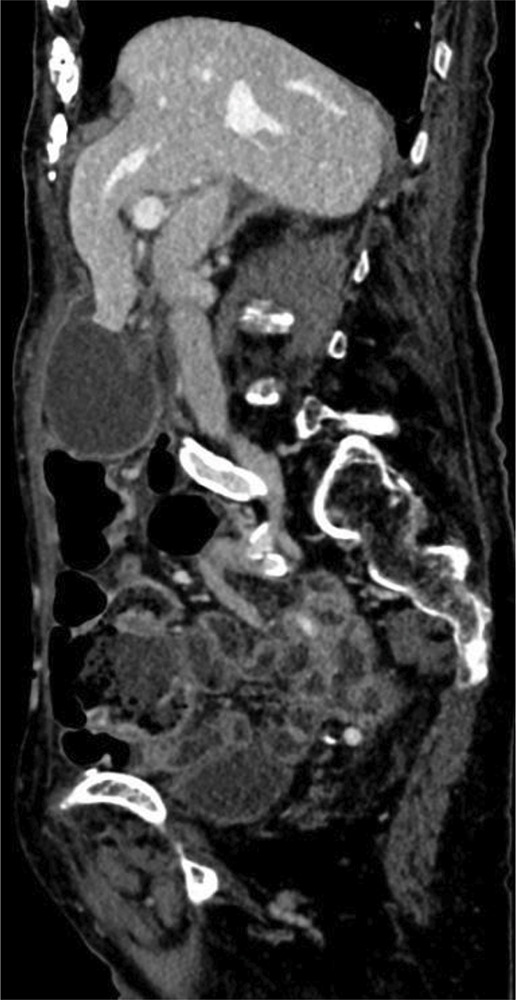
Sagittal image of a CT scan during the portal phase showing distended gallbladder inferior to the liver with “whirl sign” involving the cystic pedicle and “beak sign” with the distended gallbladder tapering at the gallbladder neck.
